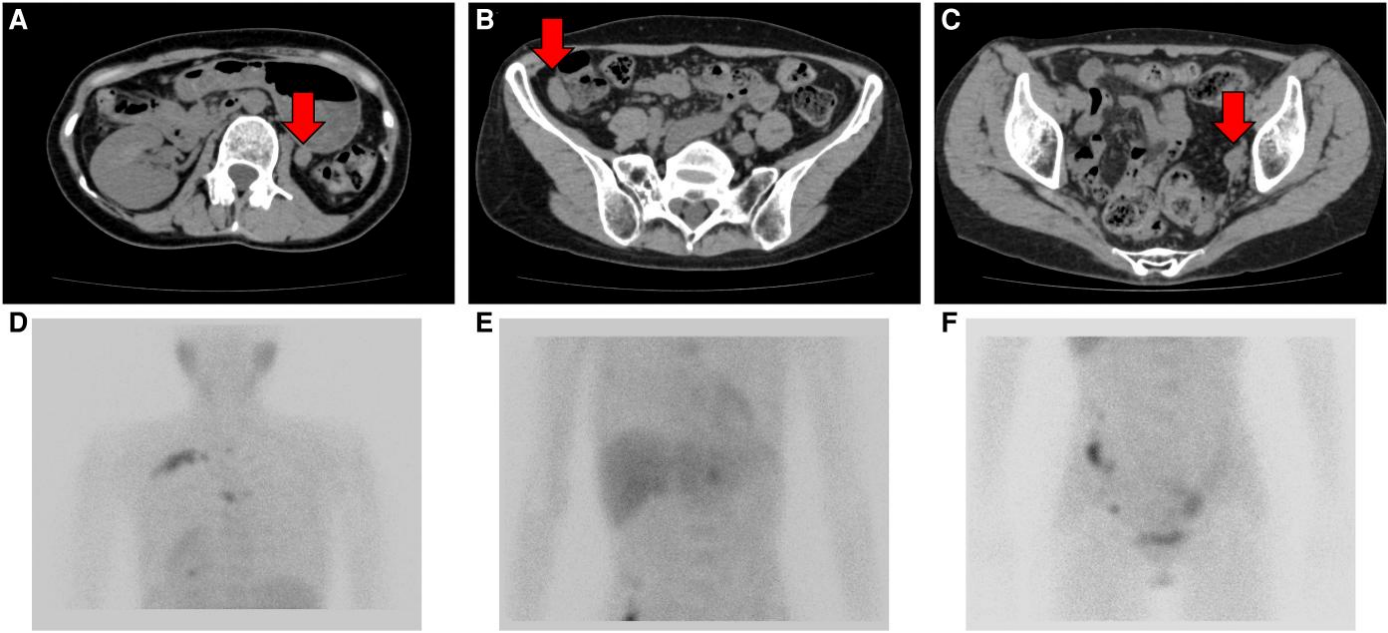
Imaging findings at the time of recurrence. CT showed multiple disseminated nodules in the abdominal cavity (A–C), and ^123^I-meta-iodobenzylguanidine (MIBG) scintigraphy showed increased accumulation in the right ribs and thoracic spine (D) and in the disseminated nodules (E, F).
